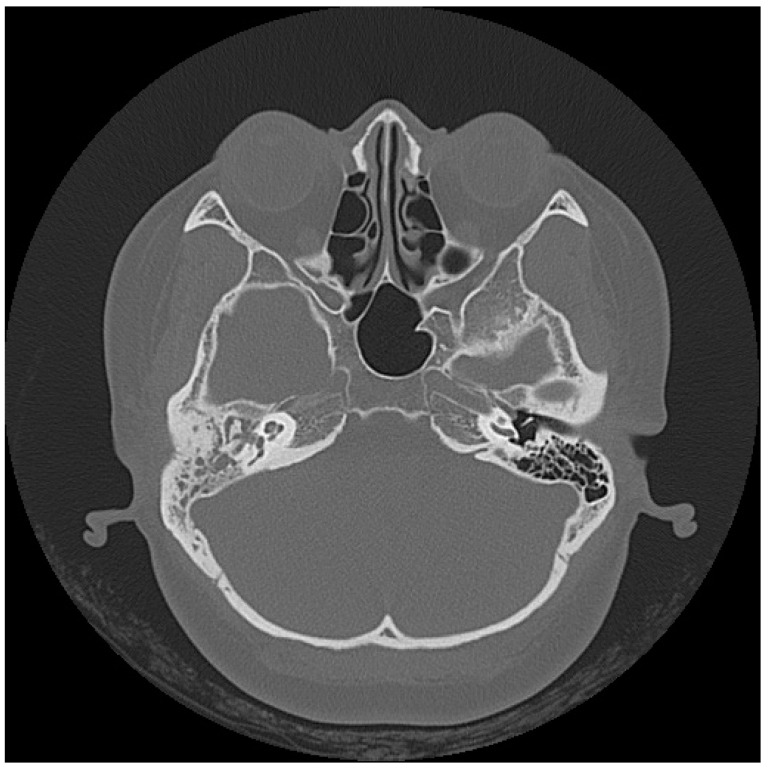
Case 5: A high-resolution computed tomography scan of the temporal bones shows diffuse opacification of the right mastoid air cells and middle ear cavity.
